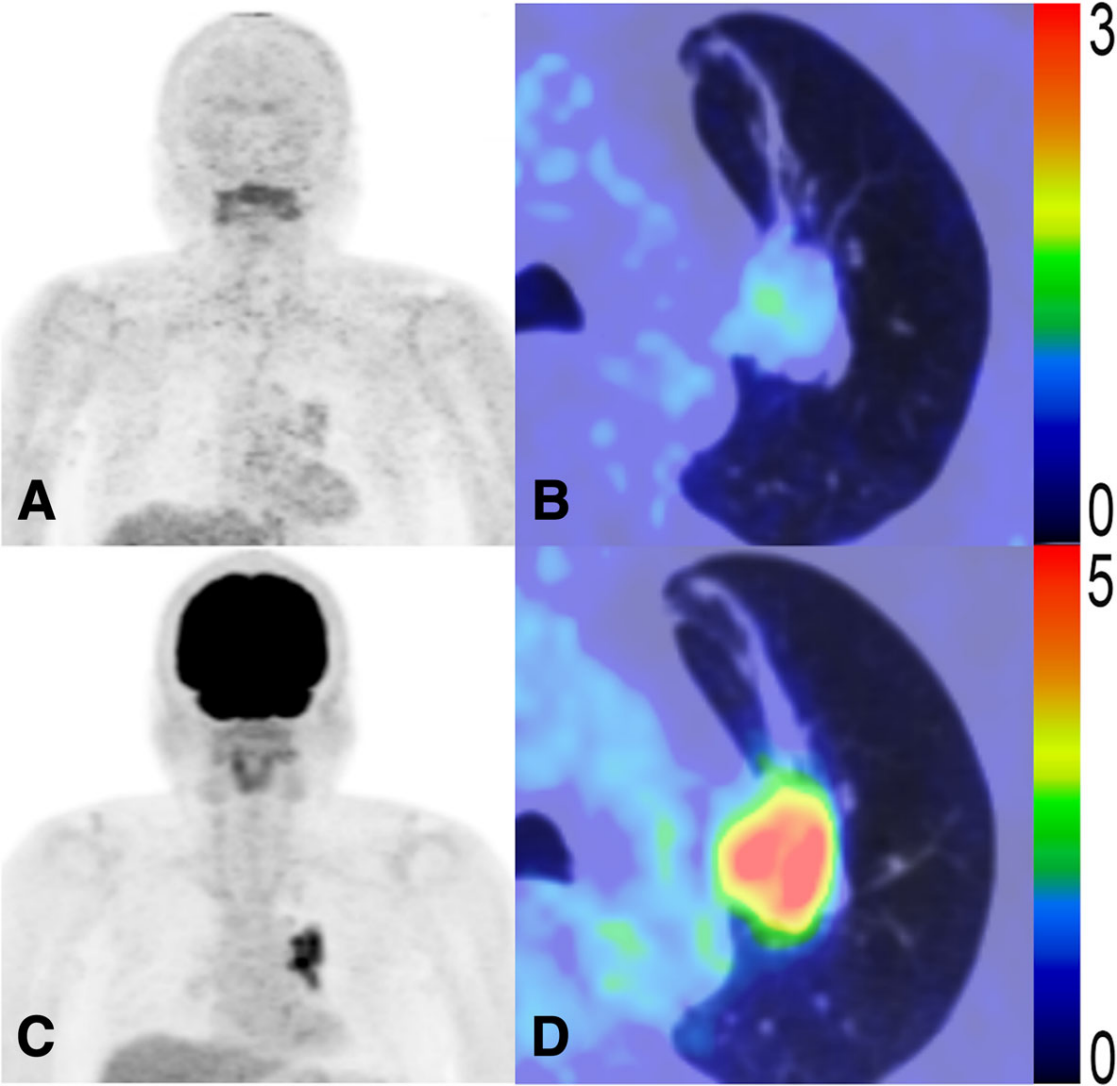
PET images of a 72-year-old male NSCLC patient at stage IIIA (T2N2M0) with low ^18^F-FAMT uptake (SUV_max_ = 1.4, MTV = 0.3 cm^3^, TLR = 0.4) (a, b) and high ^18^F-FDG uptake (SUV_max_ = 8.8, MTV = 36.0 cm^3^, TLG = 143.7) (c, d). This patient was treated with surgery combined with chemotherapy and died 1875 days later
